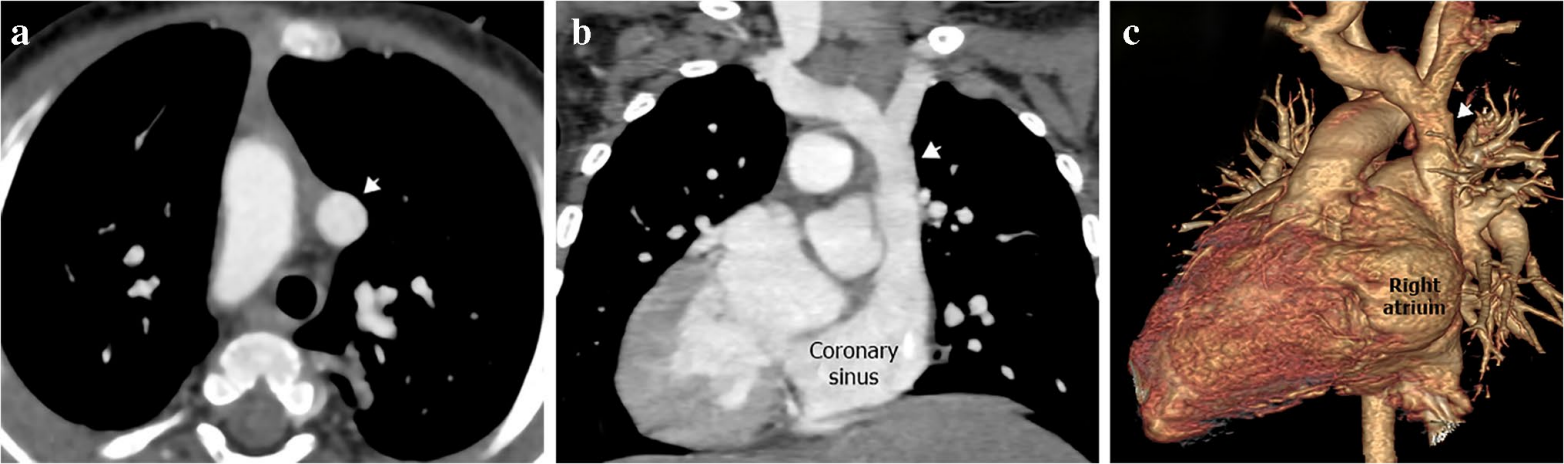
A single left superior vena cava (SVC) mirror image in a 3-year-old boy with situs inversus totalis. a–c Axial computed tomography (CT) image (a), coronal oblique CT image (b) and volume-rendered CT image (c) show a single left-side SVC (*arrows*) draining into the left-side right atrium
